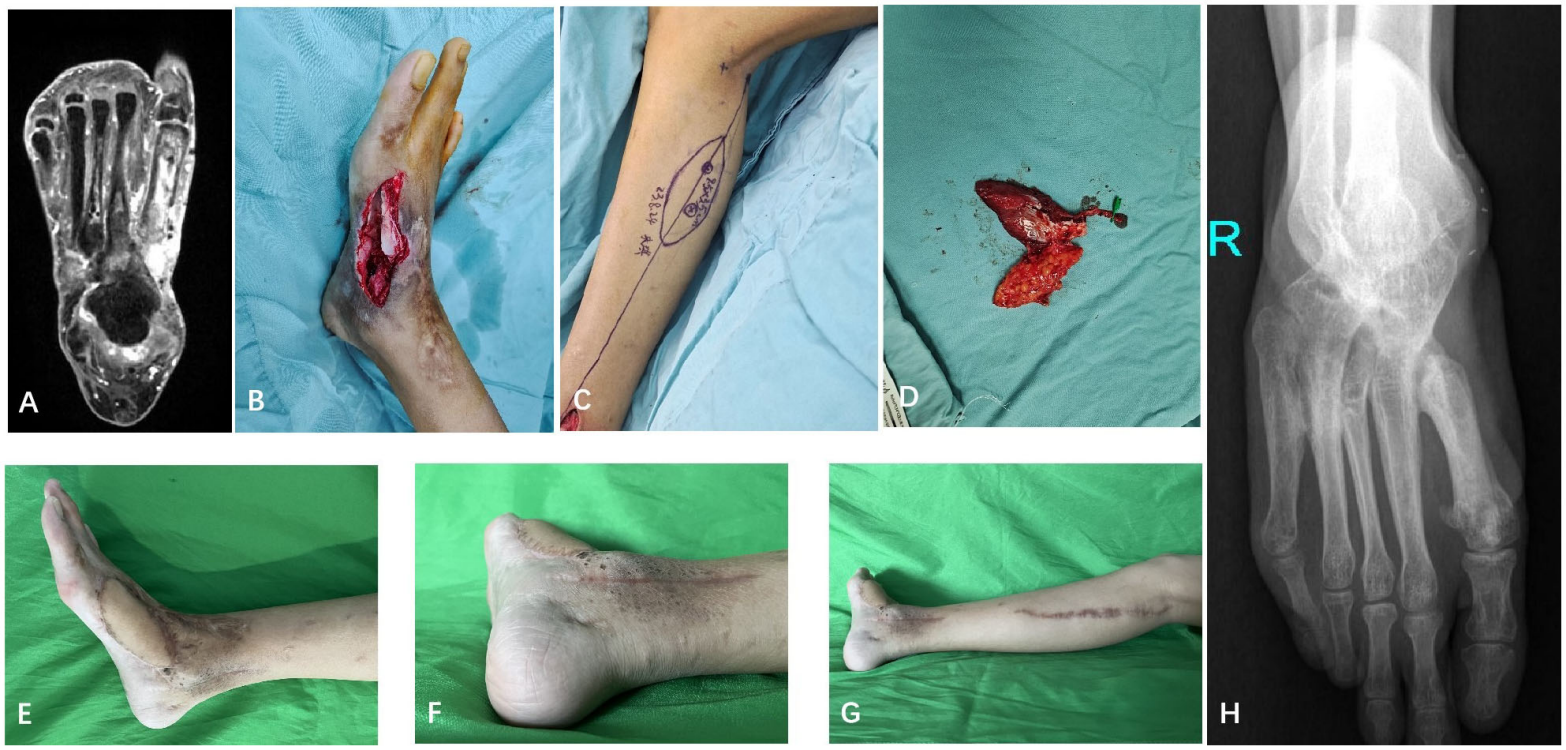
A 14 years old girl (case 5) suffered a chronic osteomyelitis in the first metatarsal. (A) Preoperative MRI manifested the diffuse infection lesion of the first metatarsal and around soft tissue, which belonged to Cinerny and Mader type IV. (B) The trepanation and partial infected bone resection were performed, the inflammatory soft tissue was debrided. A moderate-sized skin defect and a dead space were left after radical debridement. (C, D) A cMASP flap was designed and harvested. (E-G) Postoperative view of the donor and recipient site at 18 month follow-up. (H) The X-ray of the last follow-up.
